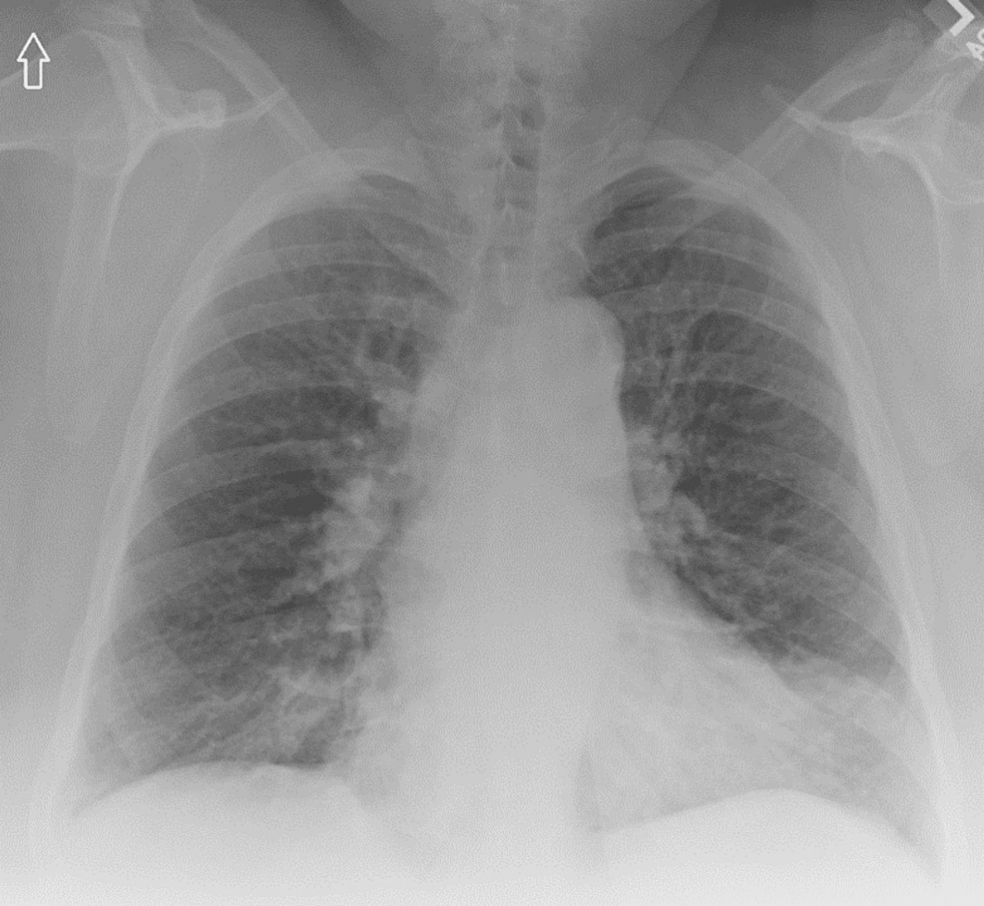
Chest radiograph on admission showing bilateral diffuse interstitial opacities.
